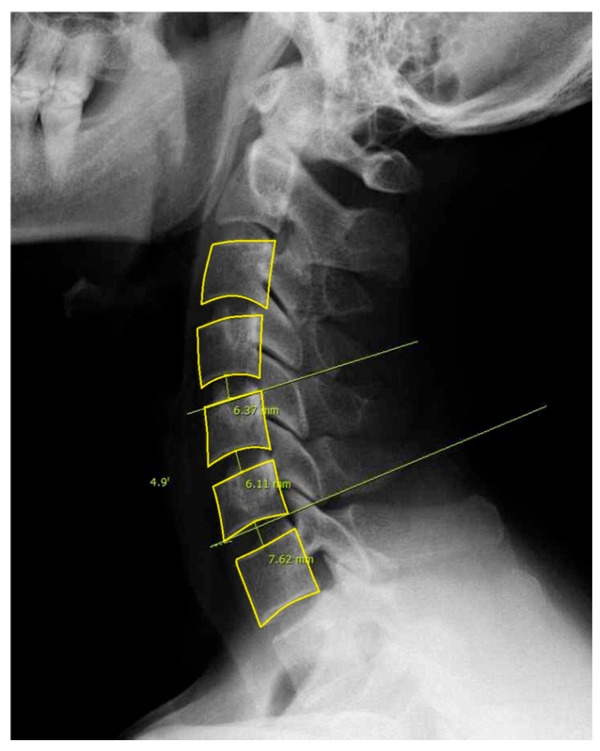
Example of labeled lateral cervical spine radiographs used in this study. The dataset includes segmentation and labeling of the vertebral bodies from C3 to C7.
